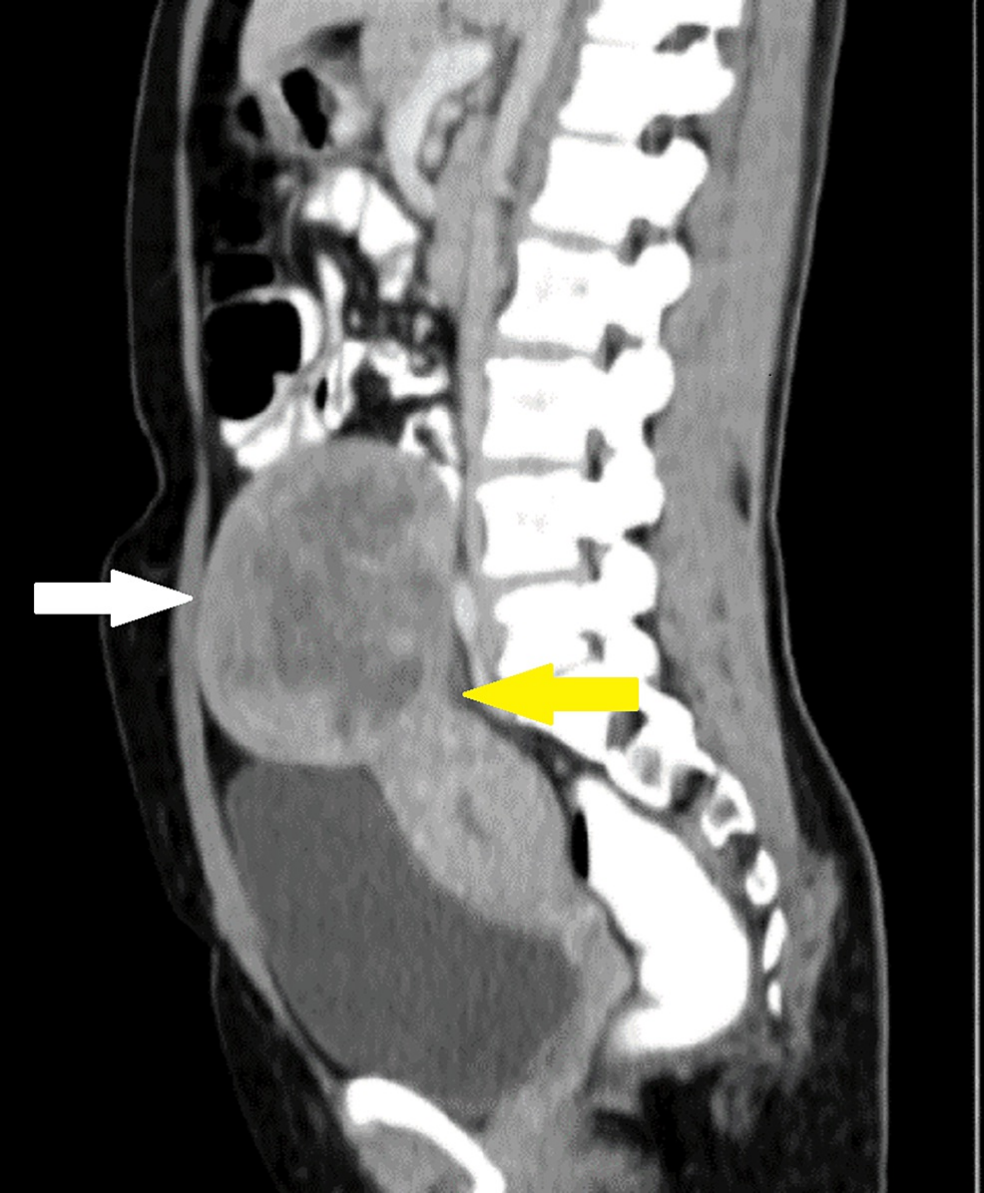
Sagittal section of a CT scan of a leiomyoma (white arrow) of size 12 x 10 x 7 cm with a stalk-like connection (yellow arrow) between the uterus and mass CT: computed tomography
